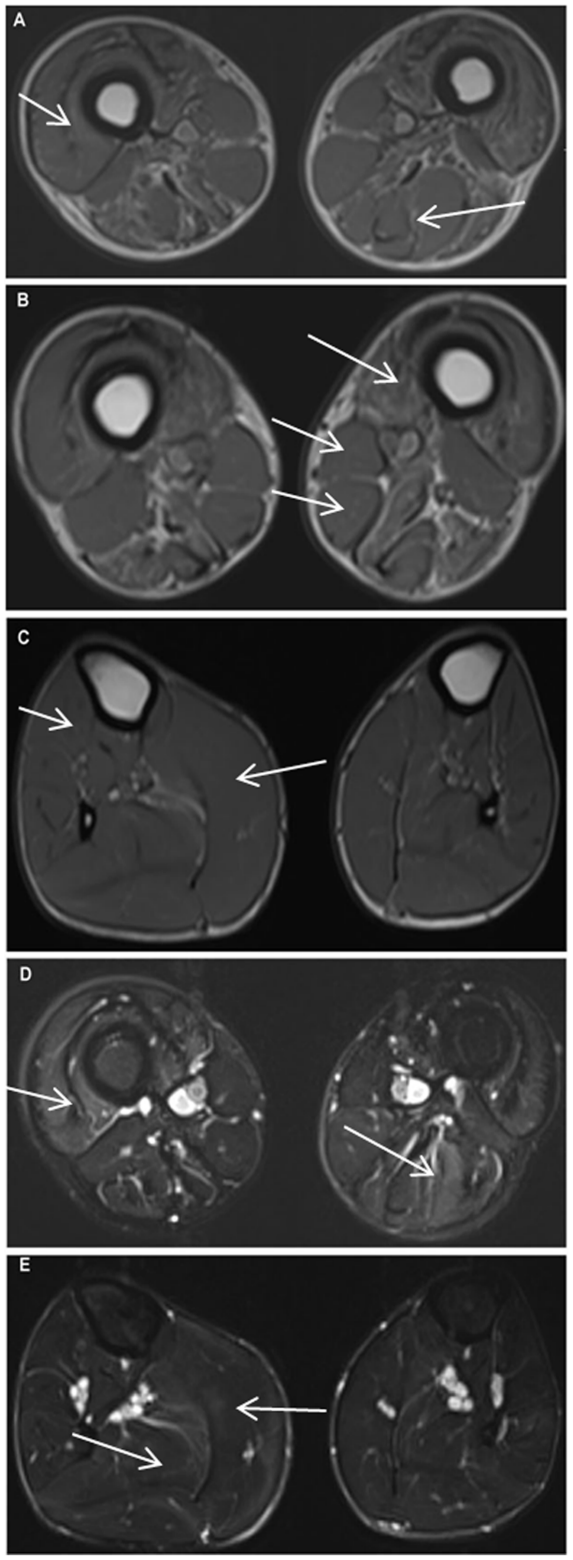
Muscle MRI of patient F97-1. A. T1W image showing global atrophy of muscles of anterior compartment, medial compartment and posterior compartment of thigh. Biceps femoris long head shows stage 3 fatty infiltration. Short head of biceps femoris, sartorius and gracilis are hypertrophied; B. shows severely atrophied vastus intermedius muscle, Mercurie stage 2b infiltration of vastus medialis muscle. Short head of biceps femoris, sartorius and gracilis are hypertrophied; C. Tibilialis anterior, extensor hallucis longus and extensor digitorum longus are atrophied (right more than left). On right side the medial head of gastrocnemius is hypertrophied and lateral head is atrophied. Left soleus is atrophied. Mild fatty infiltration of soleus muscle is noted in left side. Striking asymmetry is present; D. STIR image of thigh shows asymmetrical myoedema pattern. On the right side there is stage 3a myoedema and on the left side it is stage 2a. There is stage 2b myodema noted in the posterior compartment on left side; E. Myoedema mainly seen in gastrocnemius and soleus muscle. Stage 2b on right side and stage 2a on left side.
